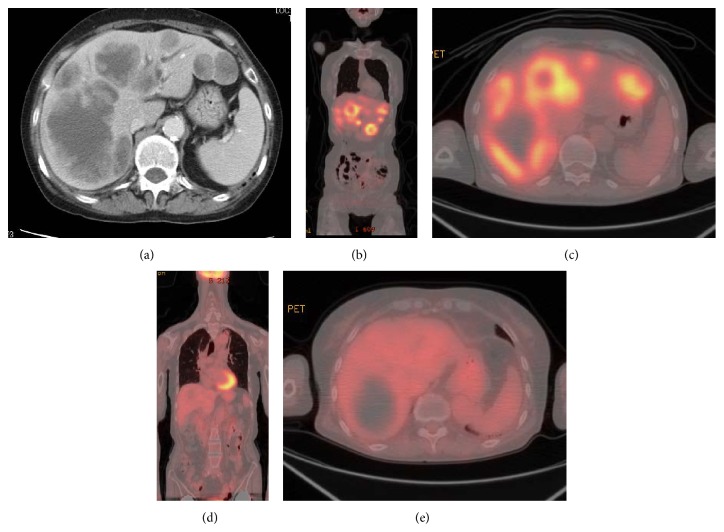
Enhanced CT images and positron emission tomography/computed tomography (PET/CT) before ((a)–(c)) and after ((d), (e)) chemotherapy.
